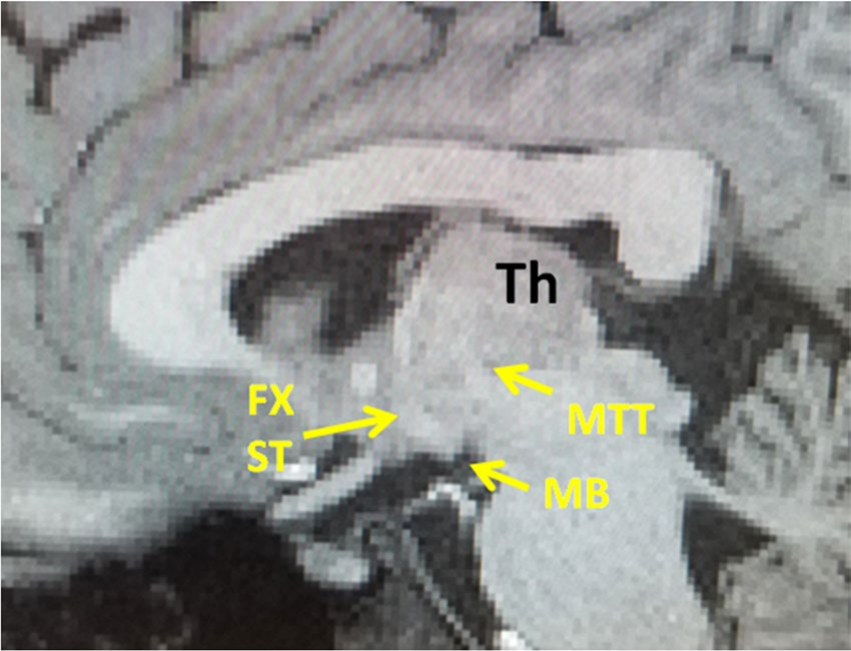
Sagittal T1 weighted MRI showing the trajectory of the MTT in relation with the precommissural fibers of the fornix and stria terminalis (Fx, ST). The MTT is visible by a T1 hypointense tract seen arising from the mammillary body (MB), projecting posteriorly and then cranially within the thalamus. The superior aspect of the MTT is not visible in this sagittal plane since the MTT projects more laterally as it ascends within the thalamus. The postcommissural fibers of the fornix and stria terminalis are visible by a T1 hypointense bundle vertically arising from the mammillary bodies.
